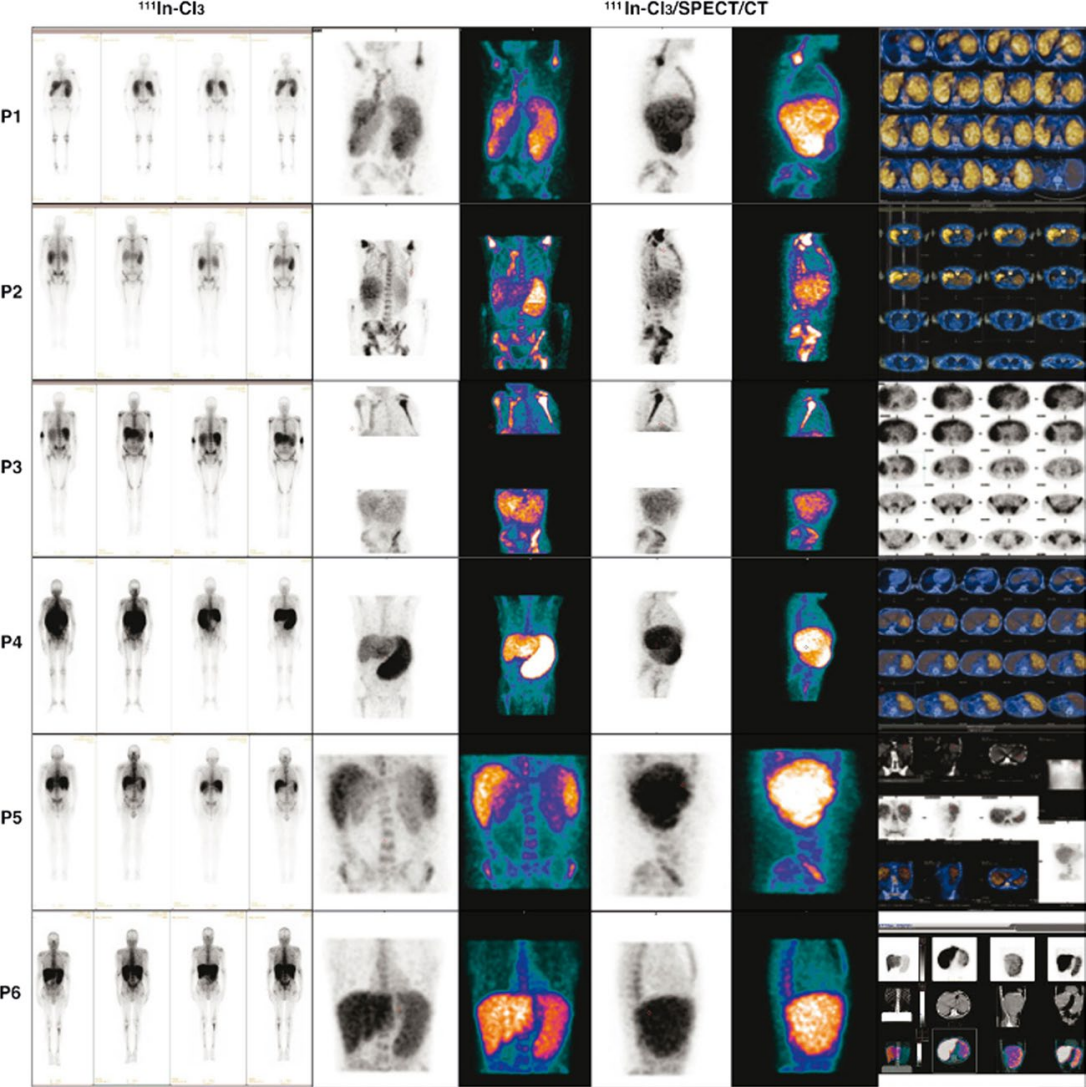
^111^In‐Cl_3_‐scintigraphy (Covidien) with single‐photon emission tomography/computed tomography acquisitions. Representative imaging of the results is shown here. UPN1 to UPN4 and UPN6 were primary myelofibrosis patients; UPN5 was a secondary (post‐ET) MF patient.
